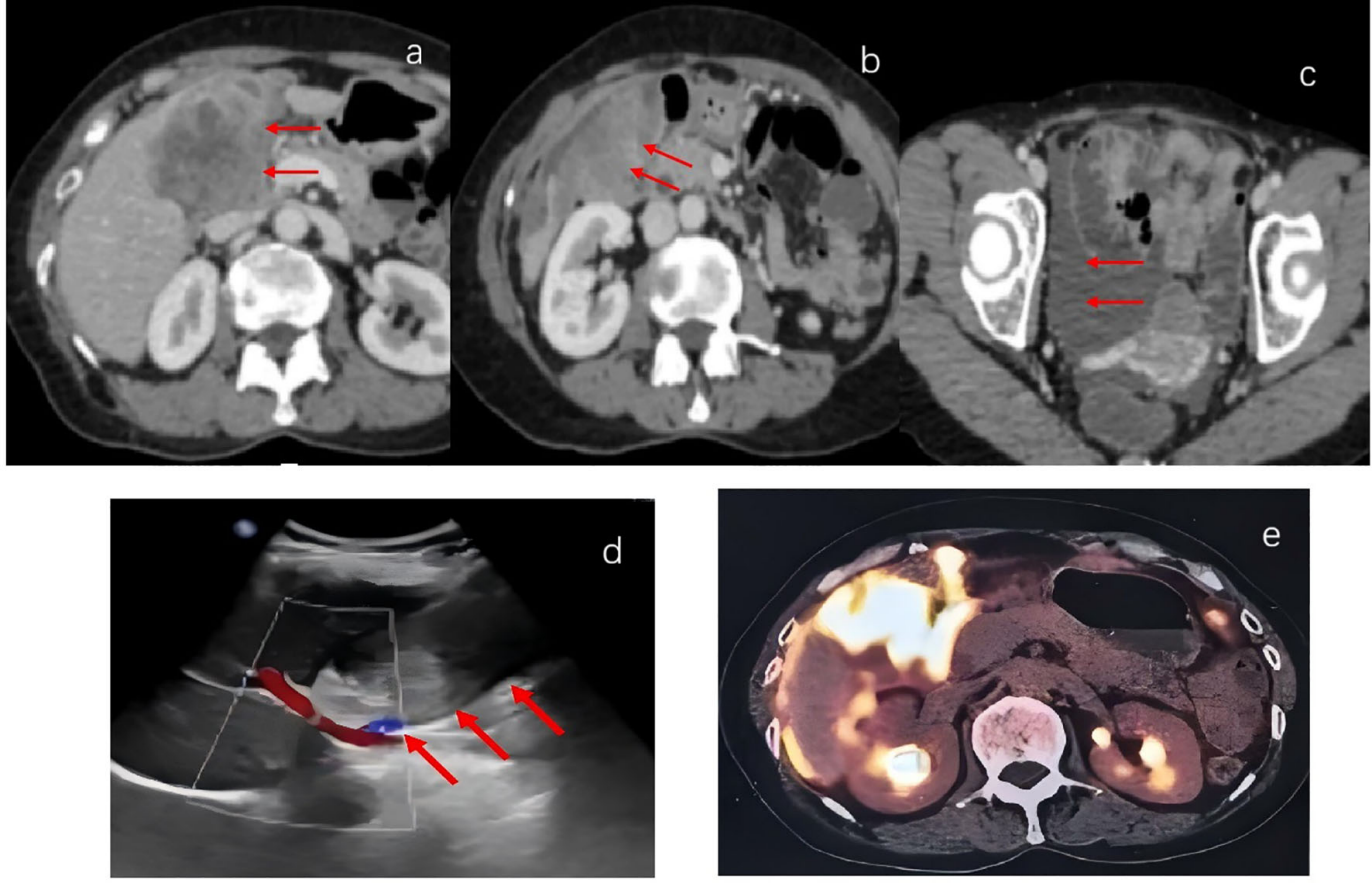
Baseline imaging before treatment: (a-c) Contrast-enhanced abdominal computed tomography (CT) scans images: A large mass was observed in the gallbladder fossa(red arrow), with invasion of the adjacent liver parenchyma and hepatocolic region; omentum thickening and multiple enlarged abdominal cavity lymph nodes, indicative of metastasis accompanied by ascites(red arrow);(d) Ultrasound images: A mass measuring 9.8 × 4.4 cm was observed in the gallbladder fossa, with an internal hypoechoic mass of irregular shape(red arrow); the gallbladder was not visible.(e) Positron emission tomography - computed tomography (PET-CT) images: the gallbladder tumor showed a maximum SUV uptake of 13.9, accompanied by peritoneal metastasis.
